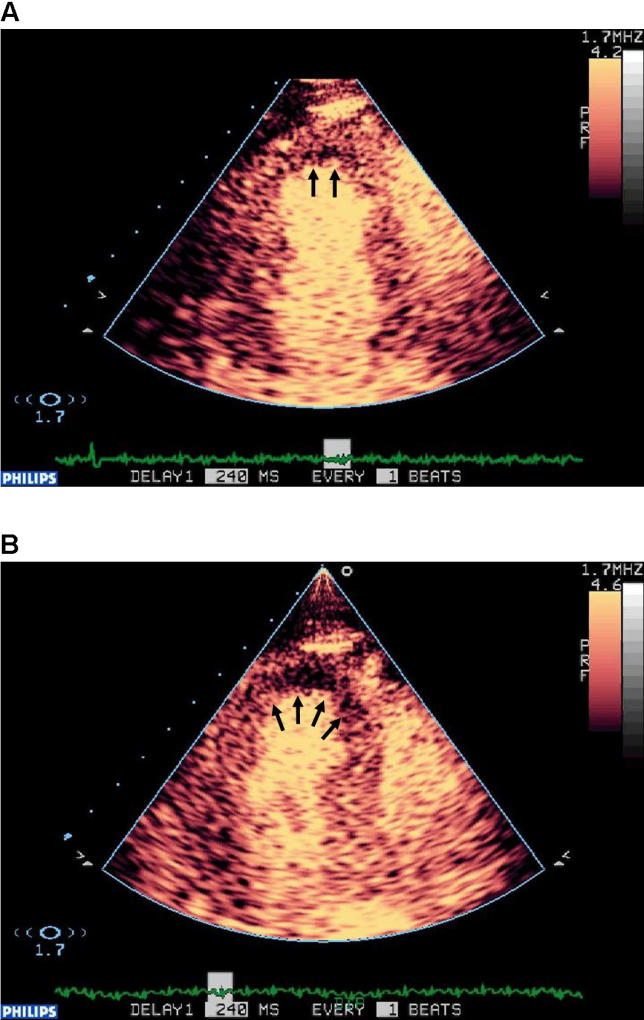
MCE: Apical Three-Chamber View Assessment (A) Apical three-chamber view assessment at rest. This assessment demonstrates a correspondingly reduced contrast uptake in the apical sub-endocardium representing an infarcted region (two black arrows) whilst the remaining myocardium shows normal opacification. (B) Apical three-chamber view assessment following stress. This assessment demonstrates markedly reduced contrast uptake in the entire septum (black arrows) suggesting mid-LAD disease.
